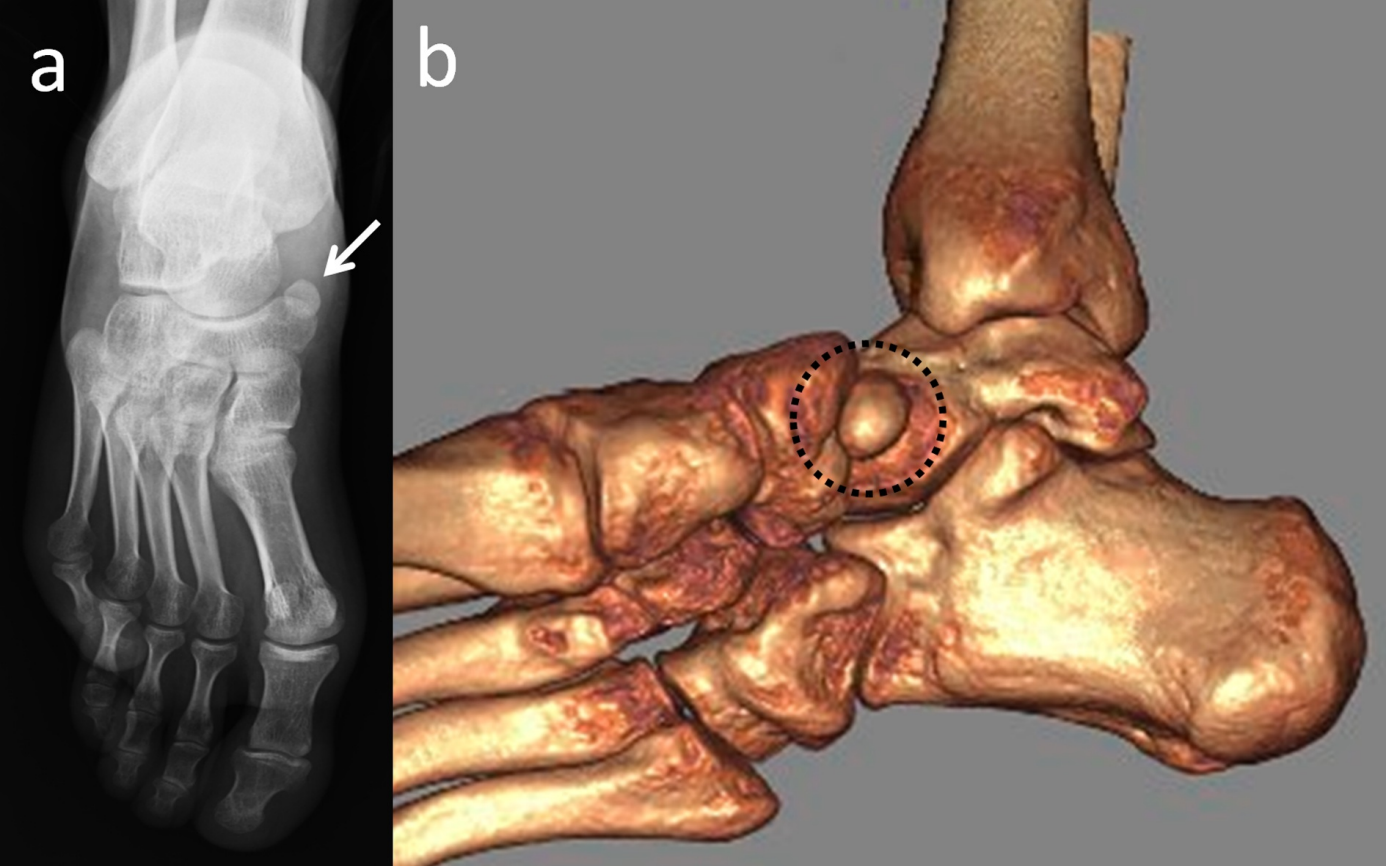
A 47-year-old male patient with an asymptomatic accessory navicular bone (a) Anteroposterior foot radiograph; (b) 3D computed tomography (CT) appearance of the inferomedial view.
